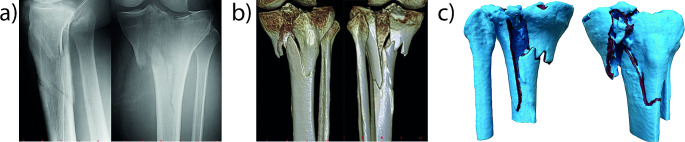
Radiograph, 3D reconstruction, and 3D print of an unusual proximal tibia fracture (Case 2)
